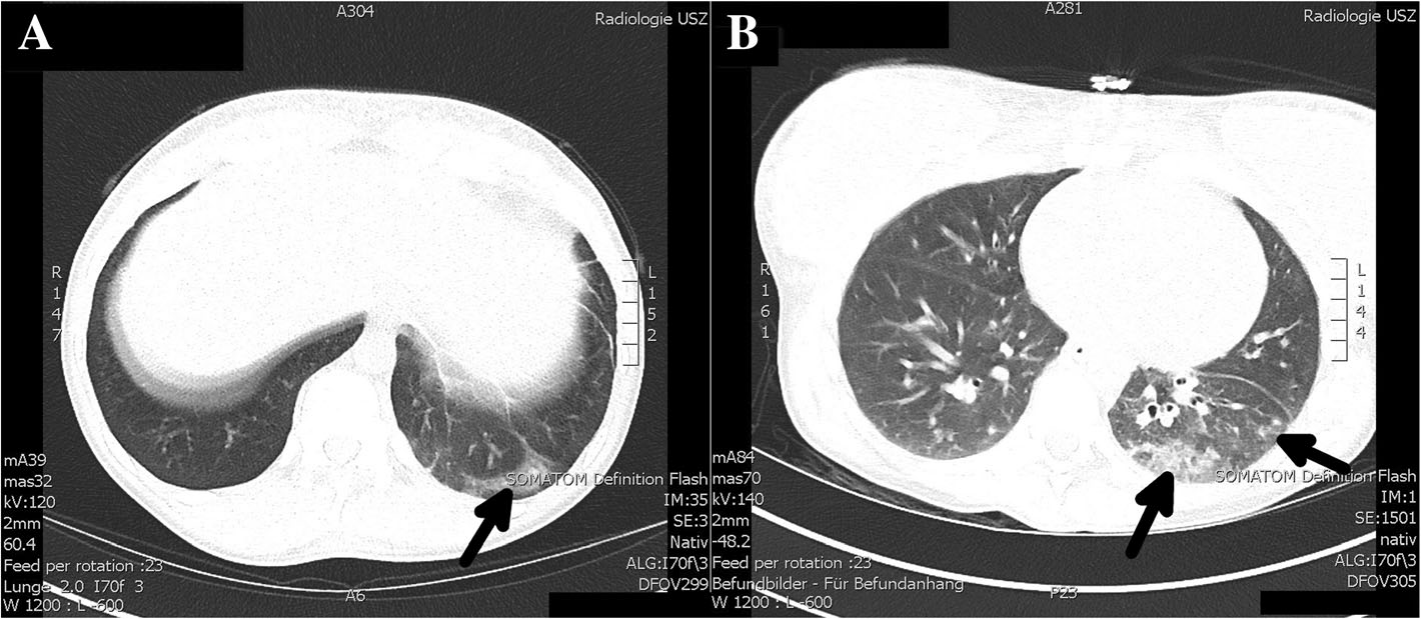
Computed tomography scans at the initial presentation (a) and 14 h later (b) showing progressive pulmonary infiltrates (arrows)
